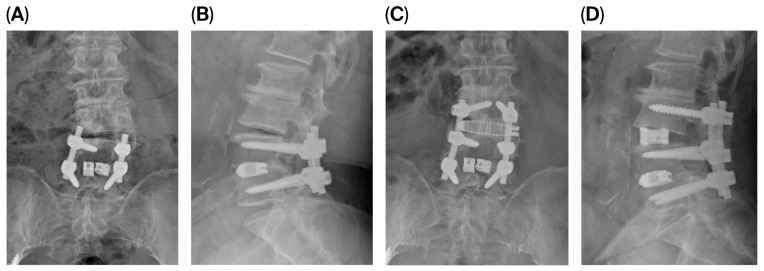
Anteroposterior (AP) and lateral radiographs before and after surgery in a representative case of prone single-position lateral lumbar interbody fusion (PSP-LLIF). (A,B) Preoperative and (C,D) 12-month postoperative AP and lateral standing radiographs of an 80-year-old female following PSP-LLIF at L3–4 with supplemental posterior instrumentation from L3 to L5. Postoperative radiographs demonstrate restored L3–4 disk height, increased lumbar lordosis (LL) and segmental lordosis (SL), and improvement in anterior disk height (ADH) and posterior disk height (PDH), with confirmed fusion and no evidence of hardware loosening, cage subsidence, or migration at 12 months.
